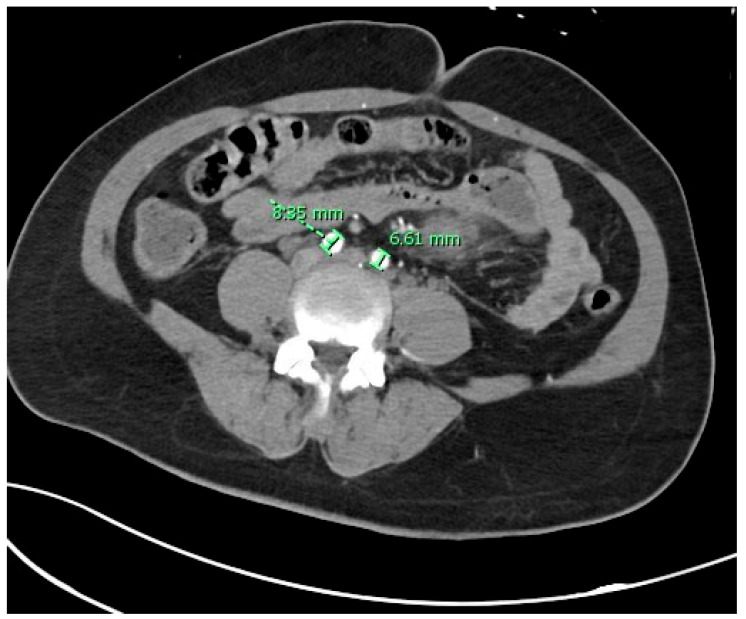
CT scan showing the measured diameter of the right and left CIA. CIA: common iliac artery.
